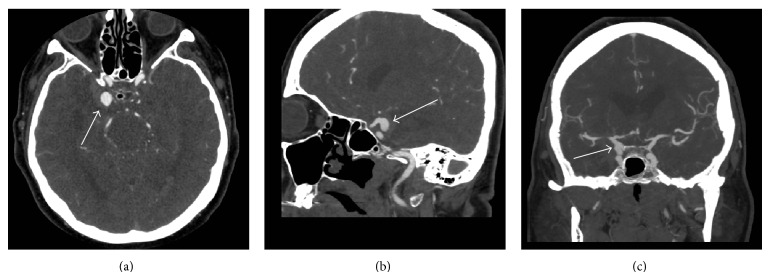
Preoperative CT-Angiogram. CT: computed tomography, CTA: computed tomographic angiography, PComm: posterior communicating artery. (a) Axial CTA displaying right PComm aneurysm (white arrow). (b) Sagittal CTA image displaying right PComm aneurysm arising from supraclinoid internal carotid artery (white arrow). (c) Coronal CTA image displaying the right PComm aneurysm (white arrow).
